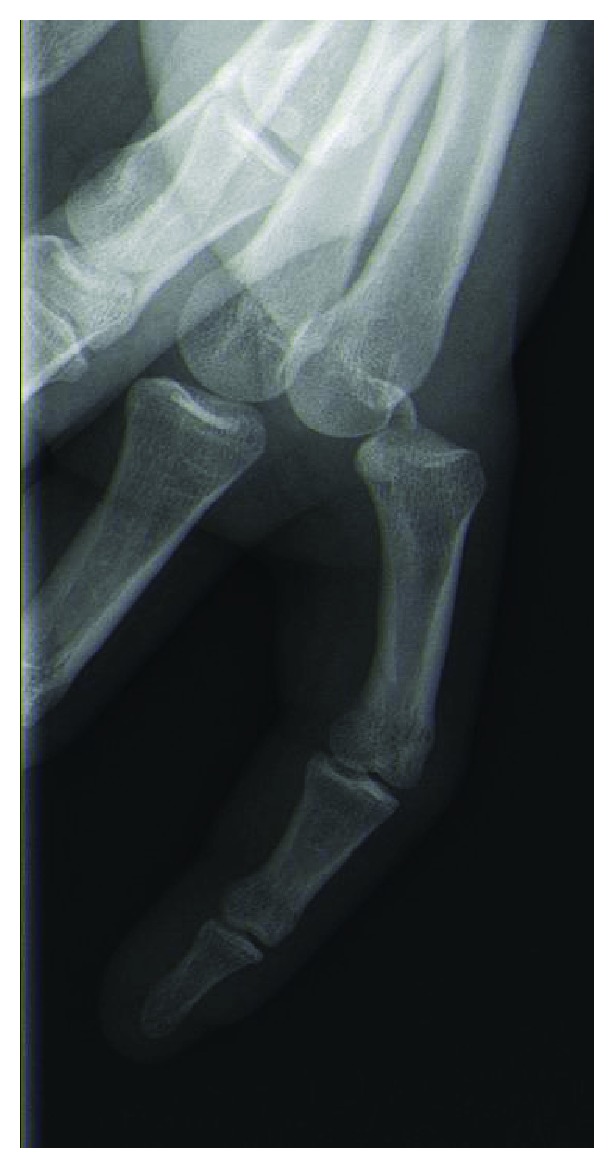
X-Ray (lateral view) showing MCP dislocation of the index finger with a small dorsal osteochondral fragment.
